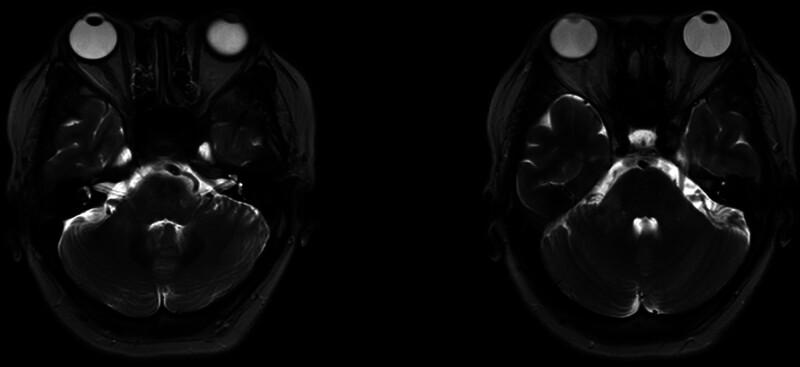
The abnormal signal in the left pontine brachium has resolved, and diminished in the right pontine brachium on T2-weighted fluid attenuated inversion recovery (T2-FLAIR) at the 4-month follow-up.
